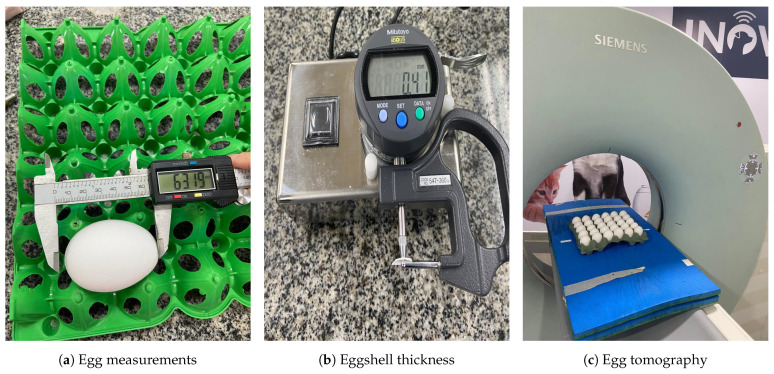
Measuring Equipment: (a) Egg measurements, length and width measured with a digital caliper (b) Eggshell thickness, measured with digital micrometer (c) Acquisition of images of chicken eggs by computed tomography.
